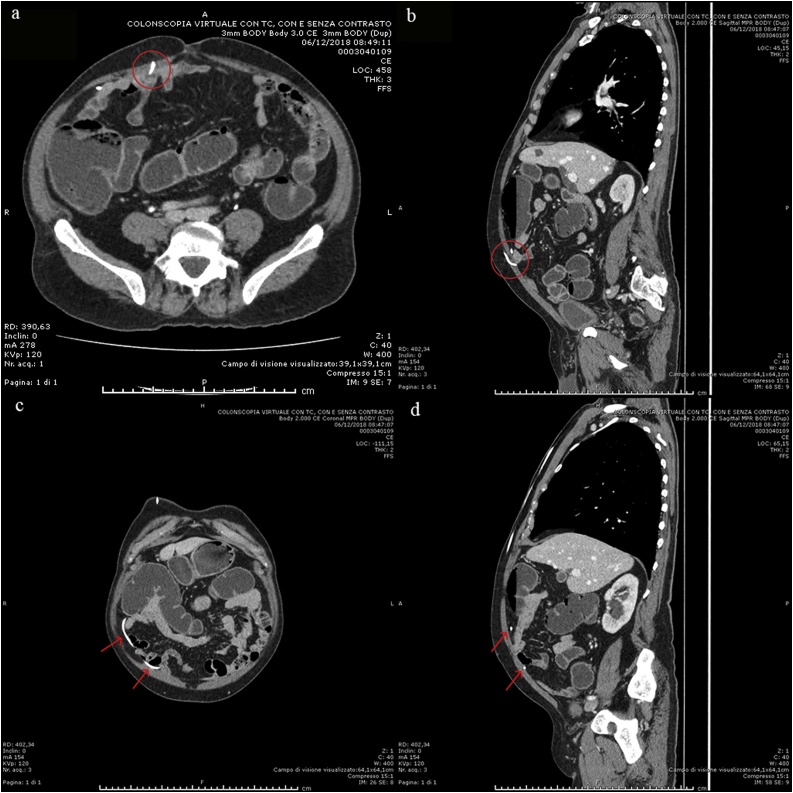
CT Scan showing the site of entrance into the abdomen (circle; a. Coronal view; b. Sagittal MPR image) and its pathway in the abdominal cavity (arrows; c. Coronal MPR image; d. Sagittal MPR image).
